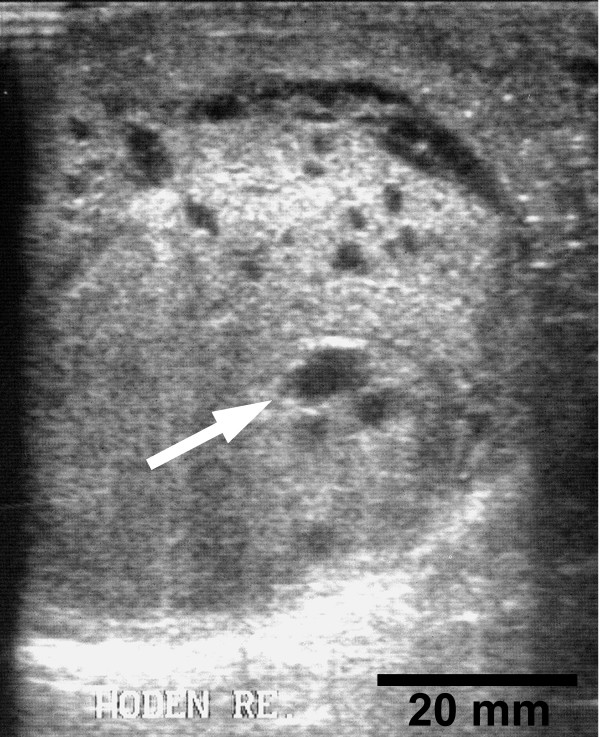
Ultrasonography, 8.0 MHz. Sagittal section of right testis showing areas of inhomogeneous parenchyma and cystic lesions (arrowhead).
